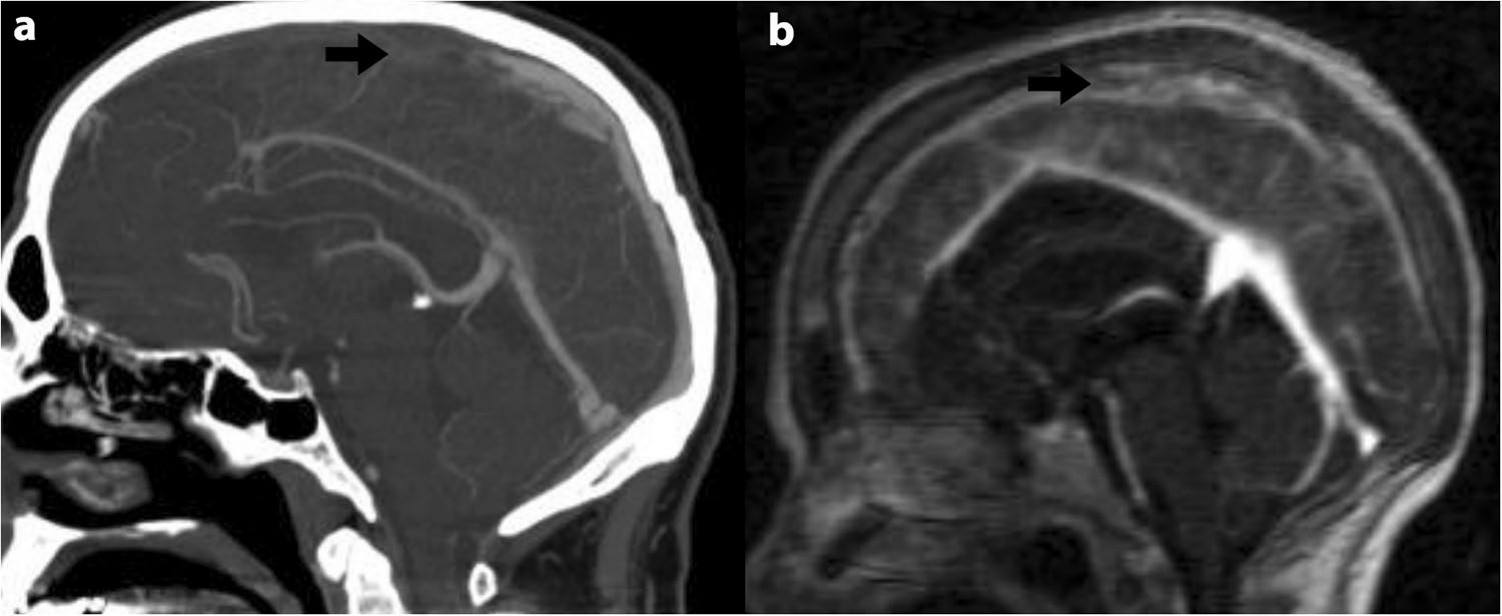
False negative CTA reading in a 38 y/o woman with CVT. The Sagittal reconstruction CTA (a) shows an irregular filling defect within the superior sagittal sinus (black arrow) that was missed by the readers. The filling defect (white arrow) is also seen in the sagittal MR venography.
